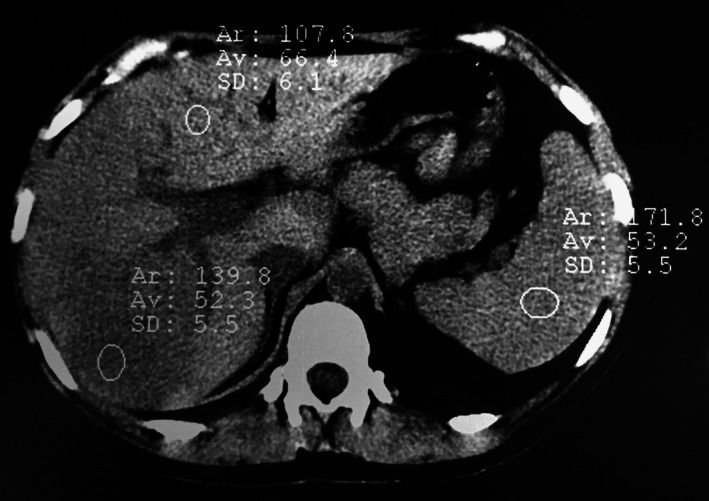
CT after delivery : the values of liver density were lower than the spleen suggesting fatty infiltration..
